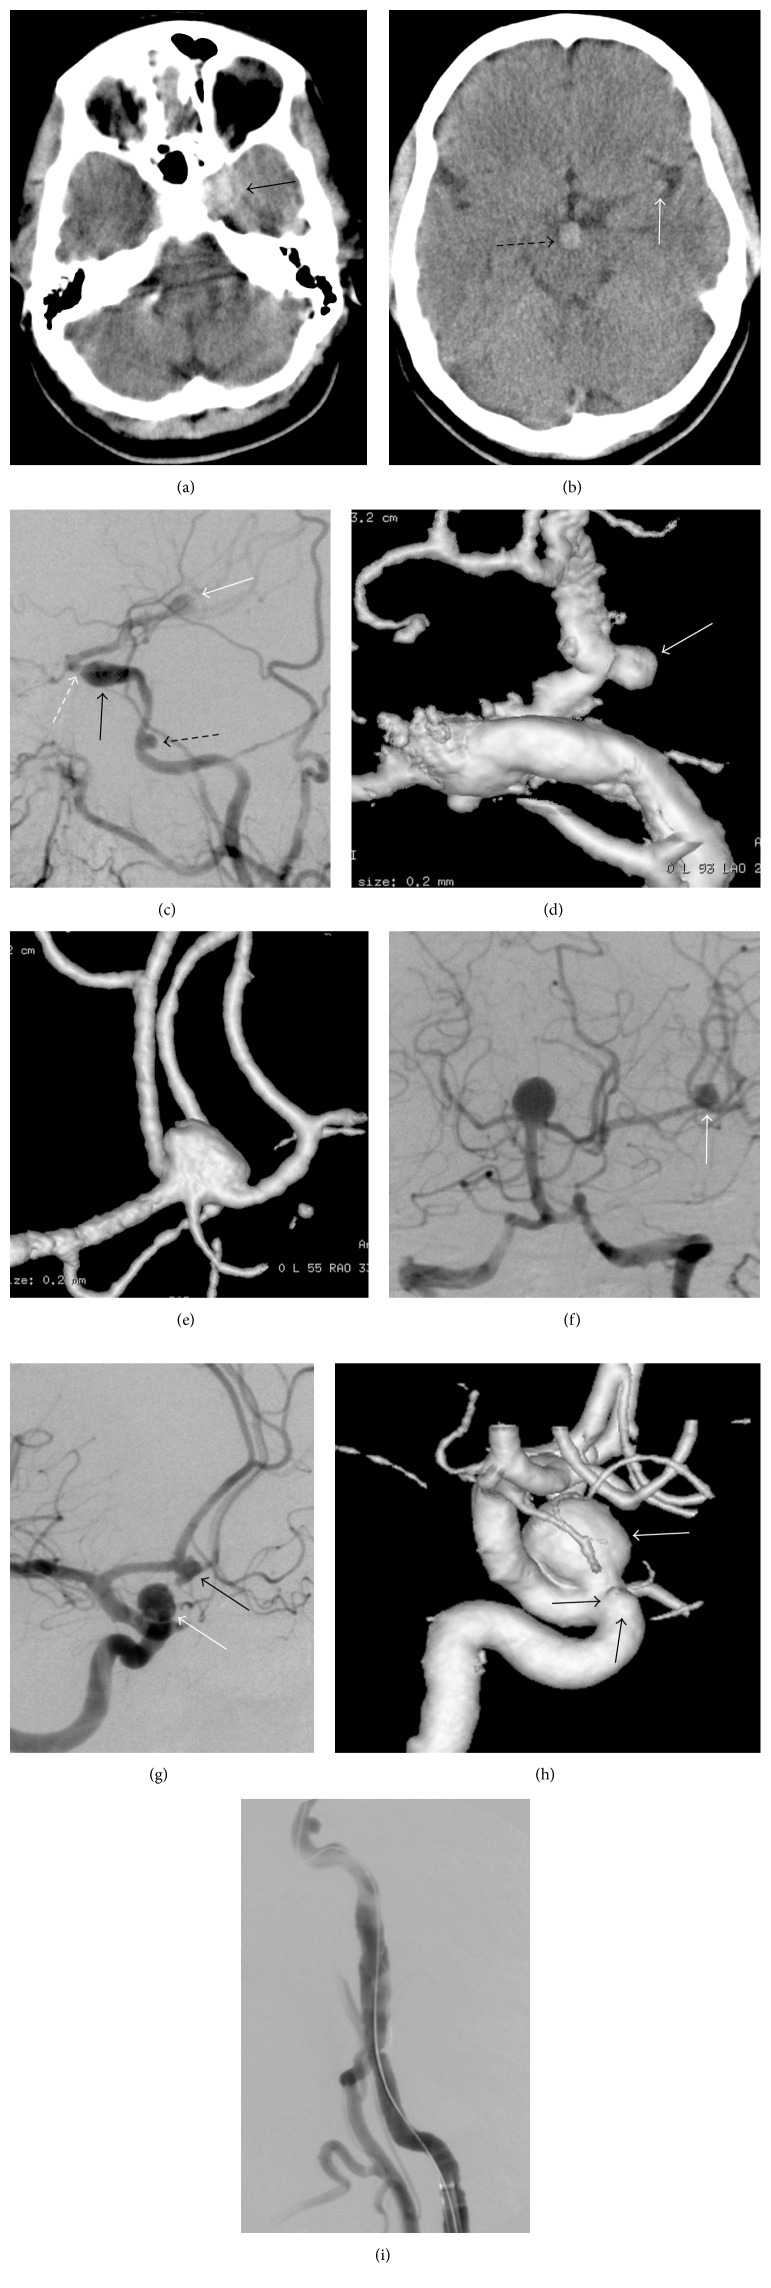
Nonenhanced axial CT slices ((a) and (b)) show three round hyperdensities: one adjacent to the sphenoid corpus on the left, another in front of the mesencephalon, and the third in the Sylvian fissure on the left, implying aneurysms of the internal carotid artery (ICA) cavernous segment (arrow, (a)), basilar tip (dashed arrow, (b)), and middle cerebral artery (white arrow, (b)), respectively. The DSA of the left carotid artery (c) reveals a small wide-necked aneurysm of the ICA lacerum segment (black dashed arrow, (c)), a fusiform aneurysm of the ICA cavernous segment (black arrow, (c)), and a middle cerebral artery bifurcation aneurysm (white arrows, (c) and (f)). A severe stenosis (white dashed arrow, (c)) adjacent to the fusiform aneurysm was also seen, resulting in reduced distal flow (c). 3D images ((d) and (e)) show the small saccular narrow-necked aneurysm of the anterior choroidal artery (white arrow, (d)) and the small saccular wide-necked aneurysm of the middle cerebral artery (e). A saccular wide-necked basilar tip aneurysm (f), a fusiform aneurysm of the anterior communicating artery (black arrow, (g)), and a saccular narrow-necked aneurysm (white arrows, (g) and (h)) and a blister-like (black arrows, (h)) aneurysm of the right ICA ophthalmic segment are seen in the DSA ((f) and (g)) and 3D (h) images. The ICA cervical segment dissection (i) possibly occurred during embolization of the aneurysms.
